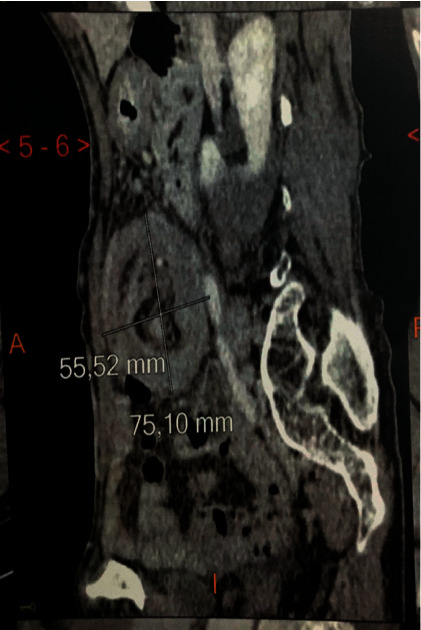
Abdominal CT scan showing large intestinal invagination (heterogeneous digestive mass).
